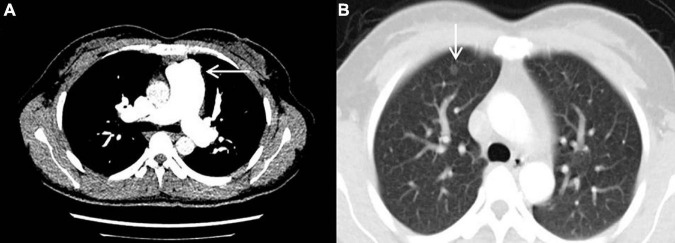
Computed tomography pulmonary angiography. (A) No obvious filling defect or vascular malformations were observed. (B) The ground-glass opacity that was 7.8 mm × 7.5 mm in diameter and located in the right upper lung lobes was seen.
